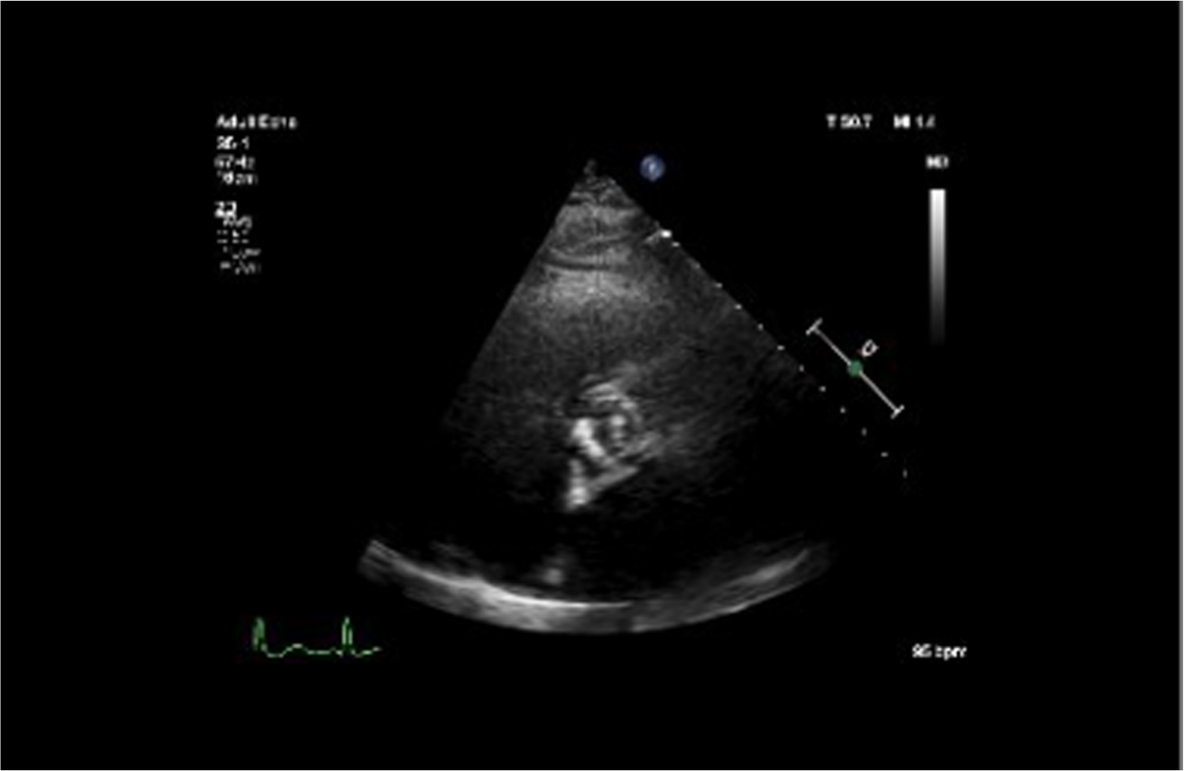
Parasternal short-axis view from the same echocardiogram
